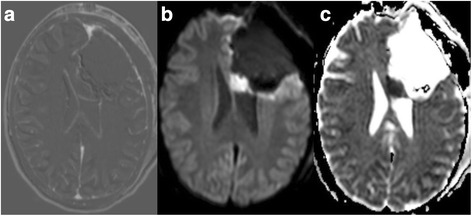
a shows a postoperative subtraction, b a postoperative diffusion-weighted image (*DWI*, b 1000), and c the corresponding apparent diffusion coefficient (*ADC*) map. Images a–c show an example of a postoperative ischemia with restricted diffusion in the genu of the corpus callosum in a patient diagnosed with an anaplastic oligodendroglioma
